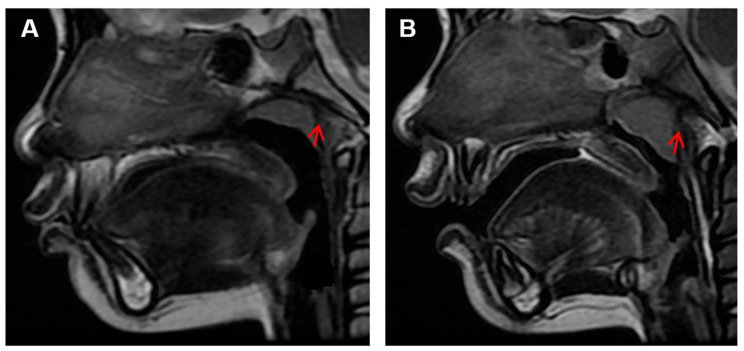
MRI Images of normal and hypertrophic adenoids. (A) The red arrow indicates a normal adenoid; (B) The red arrow indicates a hypertrophic adenoid.
